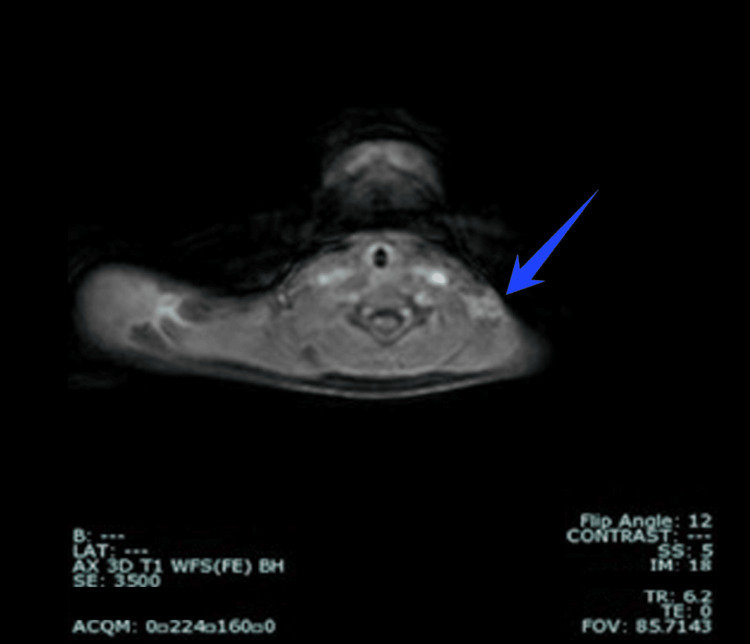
Axial post-contrast T1-weighted fat-saturated image of the neck Axial post-contrast T1-weighted fat-saturated image of the neck showing mild subtle peripheral rim enhancement to the oval-shaped multiloculated cystic lesion in the left posterior triangle of the neck.
